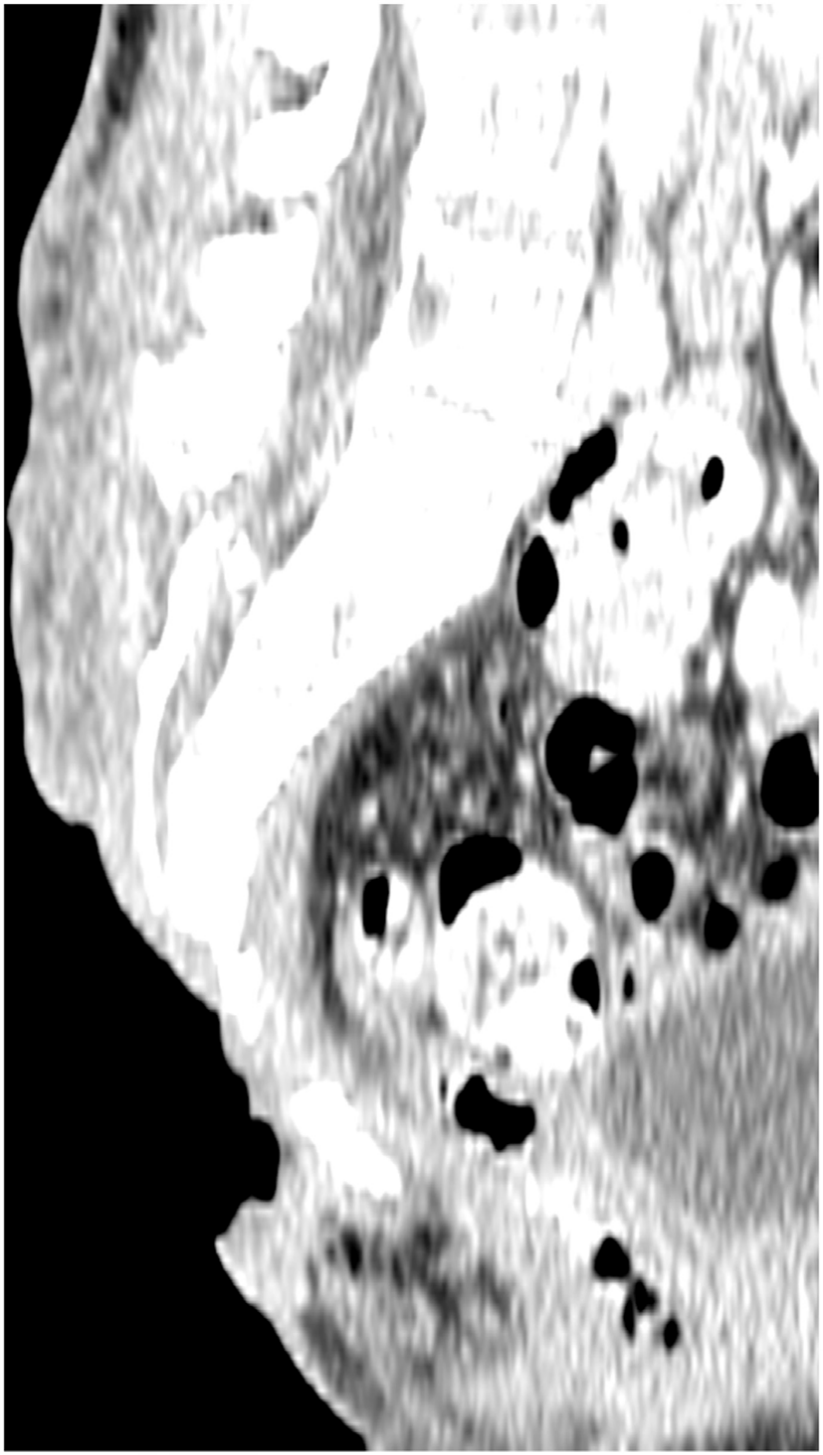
Preoperative computed tomography scan, showing the three-dimensional nature of a sacral defect.
